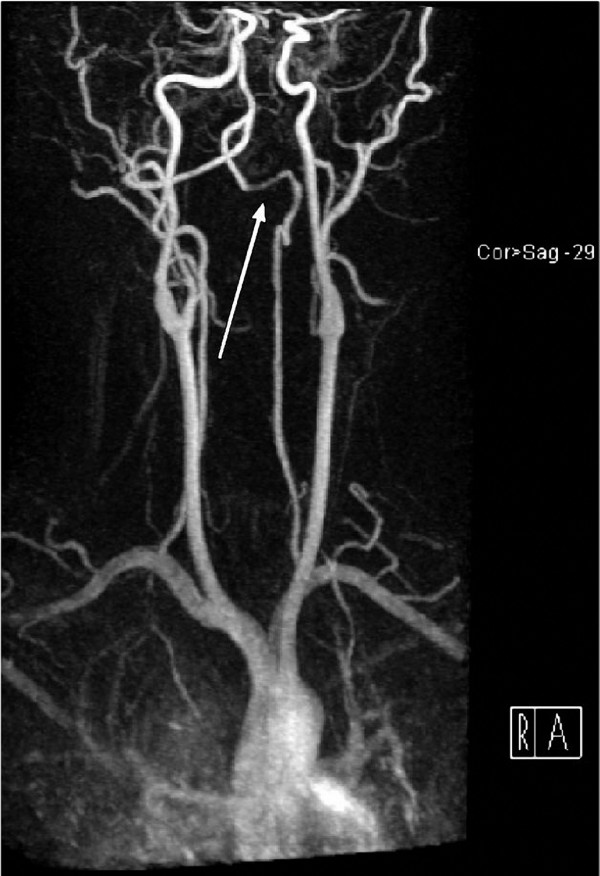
Magnetic resonance imaging maximum-intensity projection with gadolinium demonstrates irregularity and decreased vessel caliber to the V3 segment of the left vertebral artery (white arrow). This is a characteristic finding in an arterial dissection.
